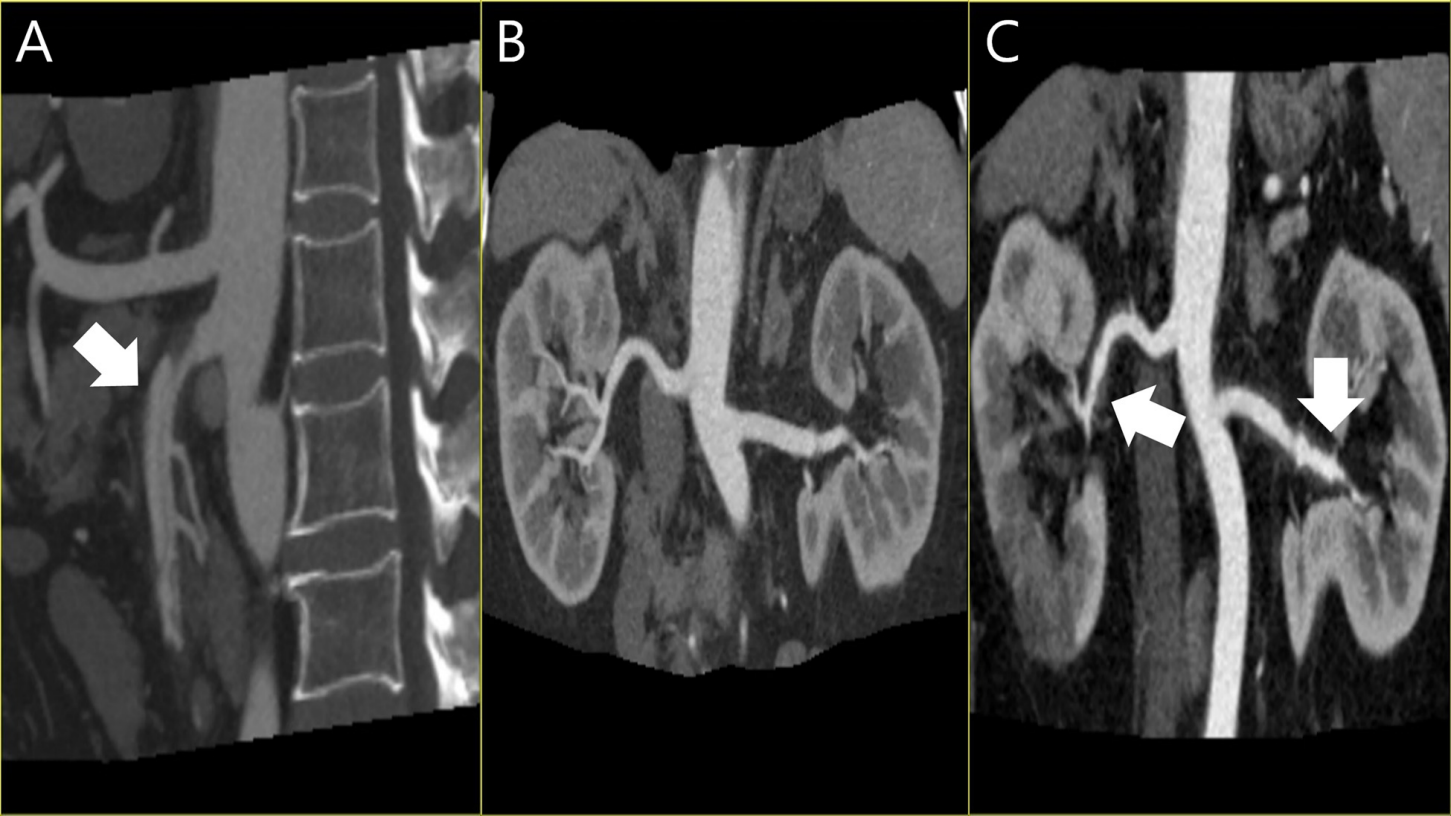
CT angiography (patient No. 2): (A & B) SMA dissection seen initially, with normal renal vasculature interpreted as symptomatic isolated dissection of superior mesenteric artery until follow-up imaging (1 week later) confirmed (C) new dissections of right and left right renal arteries with ectasia.
